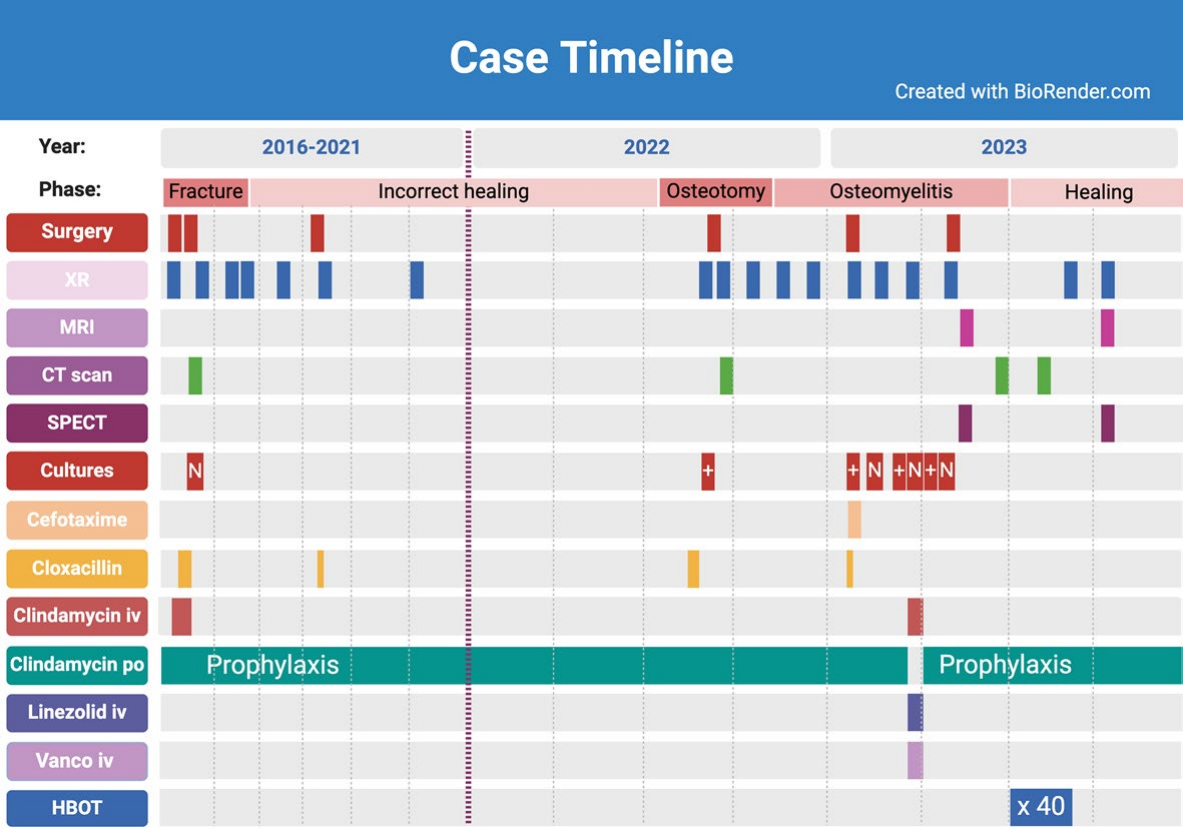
Case timeline. *XR* X-ray, *MRI* magnetic resonance imaging, *CT scan* computed tomography scan, *SPECT* single-photon emission computed tomography/computed tomography, *vanco* vancomycin, *HBOT* hyperbaric oxygen treatment, *+* positive culture, *N* negative culture
